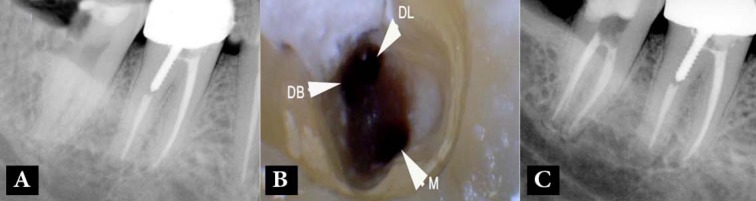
Case-1; *A)* Pre-operative radiograph shows the mandibular second molar with two separate roots; *B)* One mesial (M) and two distal (DB: distobuccal, DL: distolingual) canal orifices; *C)* Post-operative radiograph; two separate distal root canals and one mesial canal
